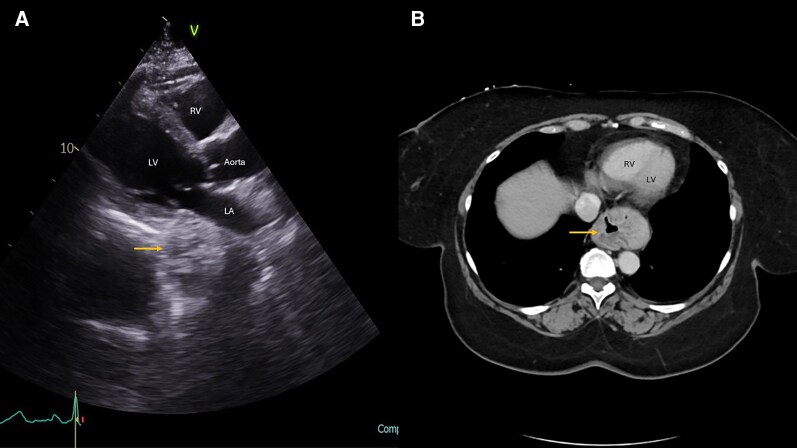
Parasternal long-axis view (*A*) showing a rounded heterogeneous echo-dense mass (arrow) flattening the posterior wall of the left atrium and appearing to be within the left atrium in the apical views (not shown). Computed tomography chest transverse view (*B*) showing the mass (arrow) posterior to the left atrium with black space indicating air. The diagnosis revealed a medium-sized hiatal hernia. LV, left ventricle; LA, left atrium; RV, right ventricle.
